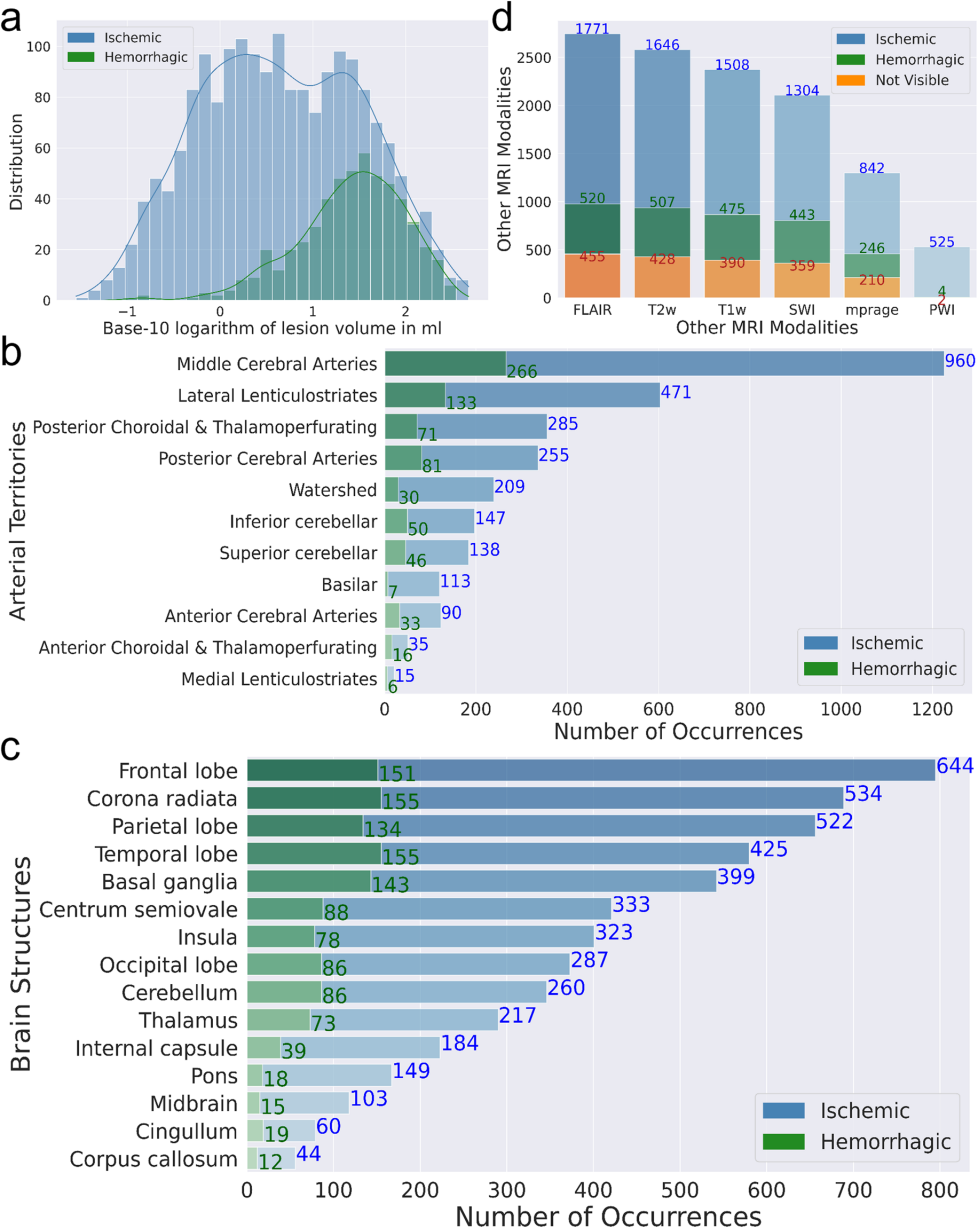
Dataset lesion and image profiles. Distribution of lesions attributed to ischemia or hemorrhage according to (a) volumes, (b) arterial territories, (c) brain structures. (d) Presence of MRI modalities other than DWI. Note that although the categorization in arterial territories is not necessarily meaningful for hemorrhage, we show it for the sake of a uniform description.
